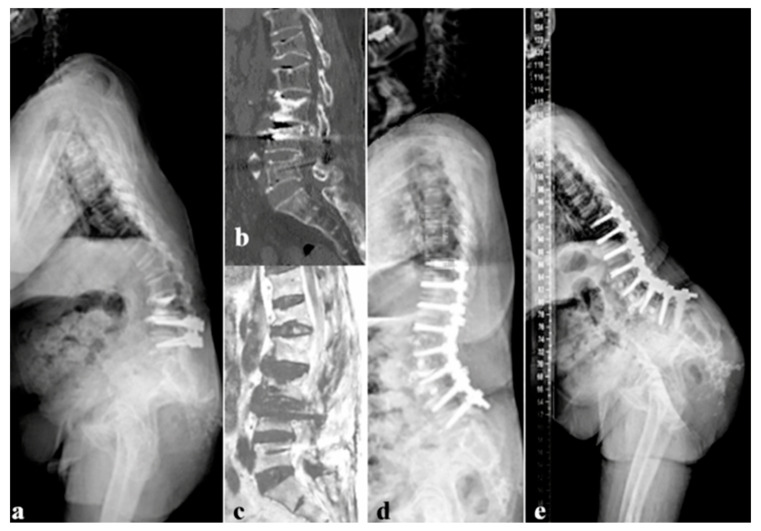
Representative case of distal junctional failure after long fusion to L5. (a) Preoperative standing lateral radiograph of a 69-year-old female patient demonstrating degenerative sagittal imbalance after vertebroplasty. SVA = 166.3 mm; PI = 54.5°; TK = −22.1°; LL = 21.5°; PT = 32.4°; SS = 21.9°; PI–LL = 76.0°; DJA = 12.4°; PJA = −15.5°. The modified Weiner grade for the L5–S1 disc was grade 0. (b) Preoperative computed tomography showing preserved L5–S1 disc height consistent with modified Weiner grade 0. (c) Preoperative magnetic resonance imaging demonstrating Pfirrmann grade C degeneration at L5–S1. (d) Postoperative standing lateral radiograph obtained four weeks after surgery demonstrating improved sagittal alignment. SVA = 60.4 mm; PI = 54.7°; TK = 21.7°; LL = 45.0°; PT = 21.3°; SS = 33.2°; PI-LL = 12.7°, DJA = 5.8° and PJA = 1.3°, however, LIV fracture developed at 3 months postoperatively. (e) Follow-up radiograph obtained four years after the index surgery demonstrating recurrent sagittal imbalance secondary to distal junctional failure.
